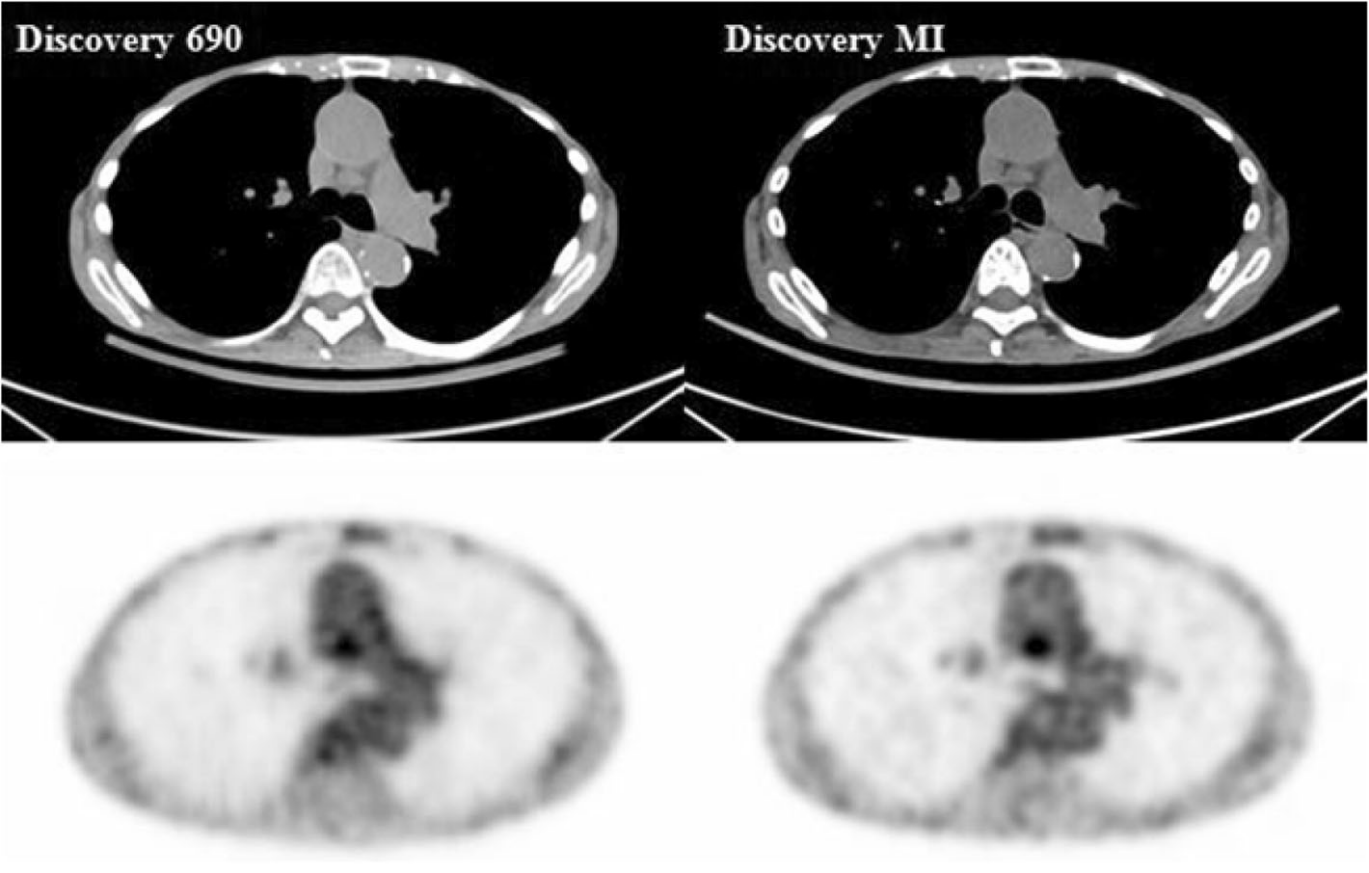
Axial PET and CT images. An example of axial PET and CT images of a mediastinal lymph node with a SUV_max_ of 2.7 on the Discovery 690 and 3.6 on the Discovery MI
